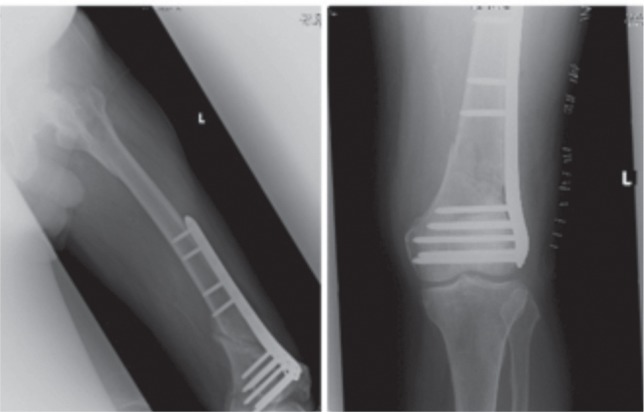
Radiographs after LISS plate insertion.
